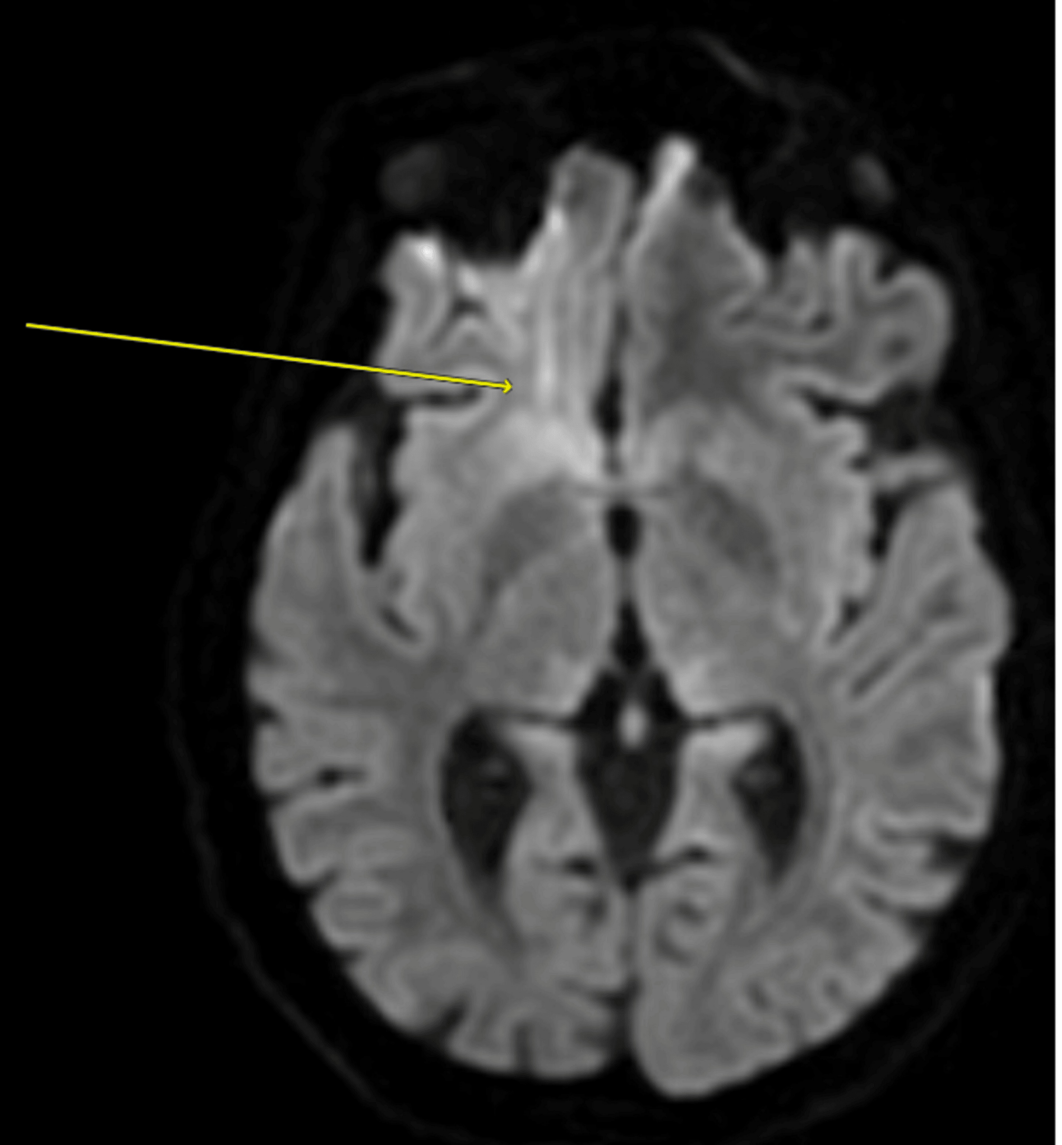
MRI scan of the brain with contrast, showing focal hyperintense signals in the right frontal subcortical region (yellow arrow) in the DWI sequence, which may suggest cerebral edema
